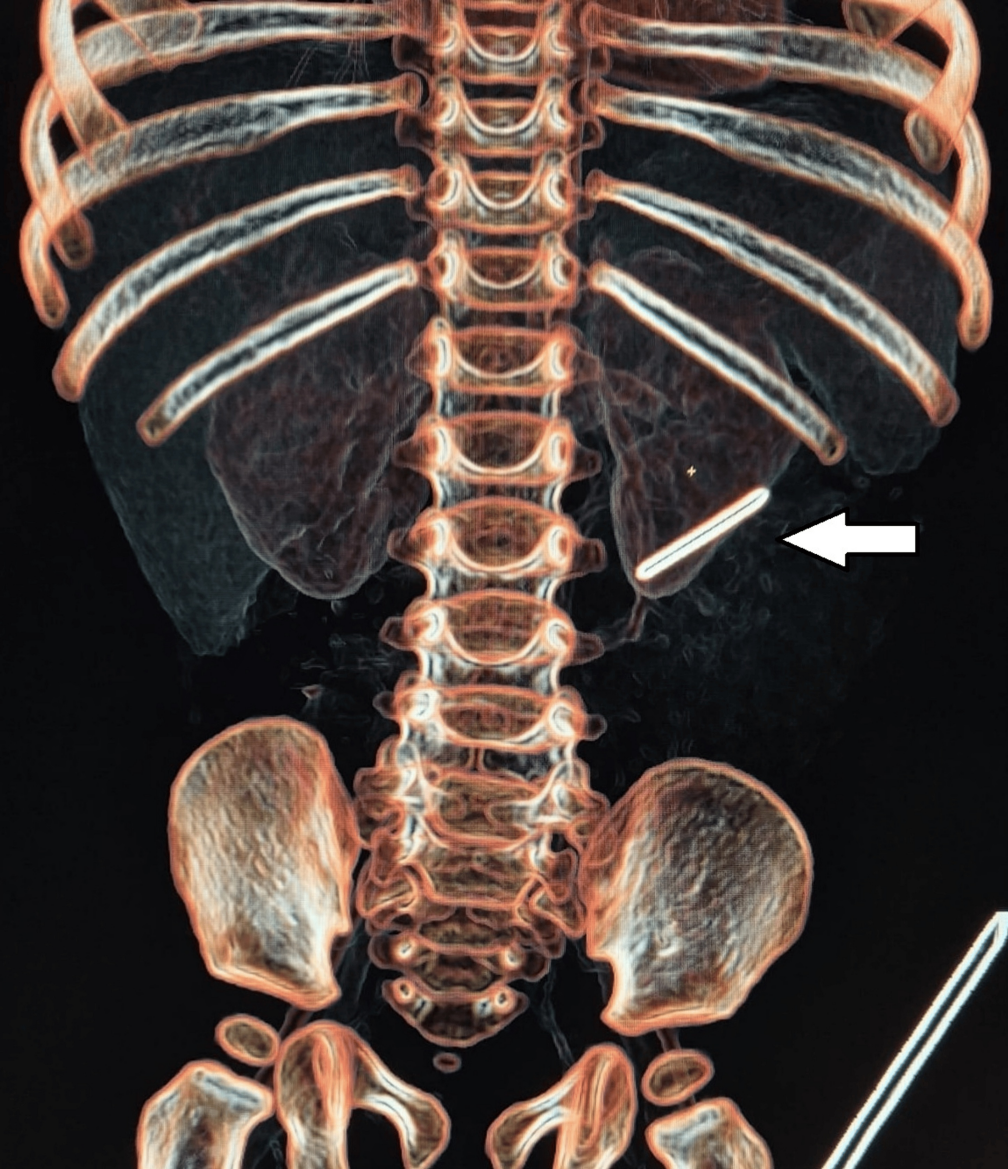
3D reconstructed computed tomography (CT) image showing a linear foreign body in the lower pole of the left kidney.
